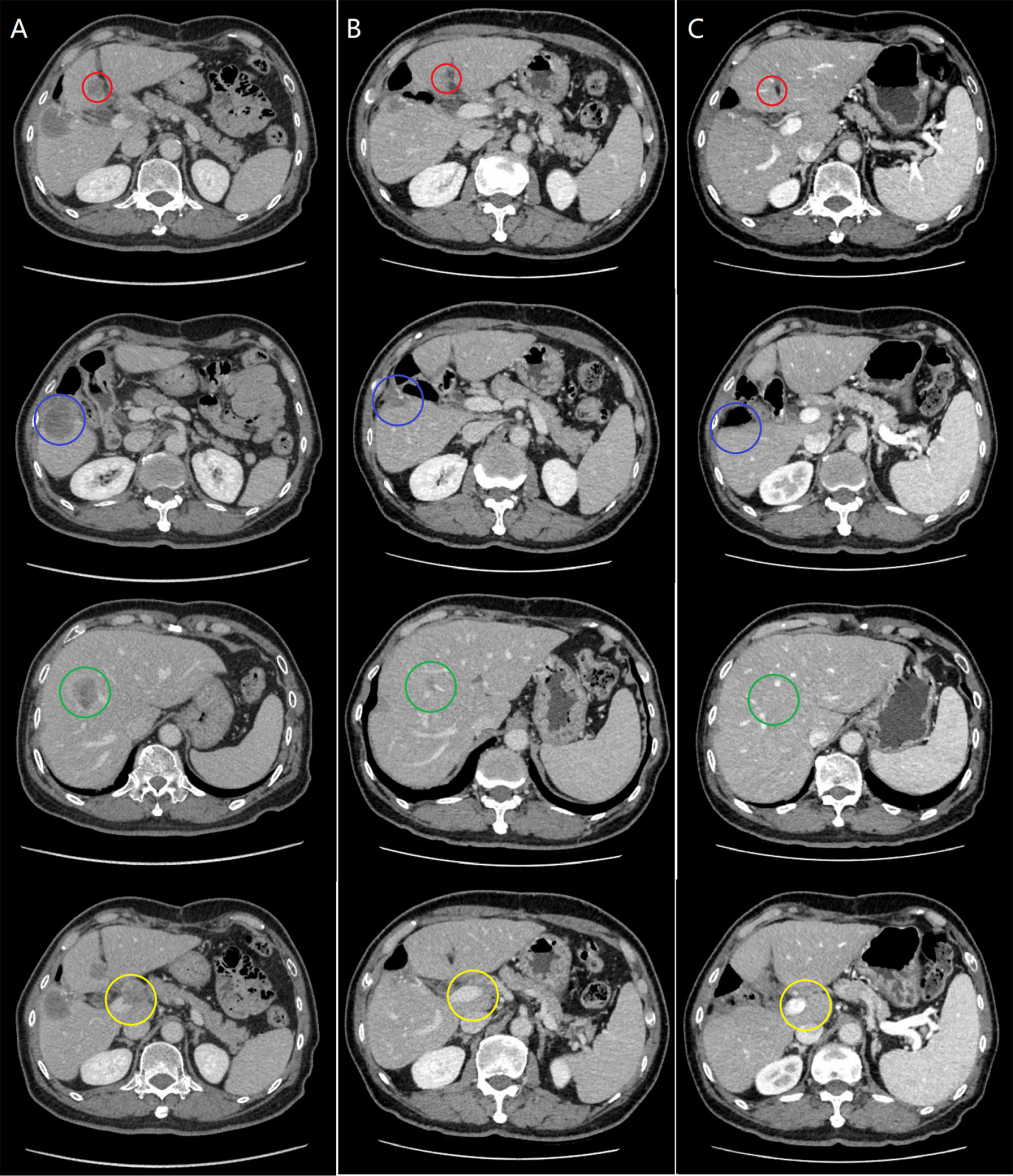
The evaluation of tumor response during the clinical course. (A) Pretreatment computed tomography (CT) revealed recurrent lesions in the segment IV (red circle), V (blue circle) and IV/VIII (green circle) of the liver, and a metastatic lesion in the hepatic hilum with tumor thrombus in the main portal vein (yellow circle). (B) After two courses of combination therapy, CT showed the intrahepatic lesions decreased in size, and both the lesion in the hepatic hilum and the main portal vein tumor thrombus disappeared. (C) After six courses of combination therapy, CT showed complete resolution of all the lesions.
